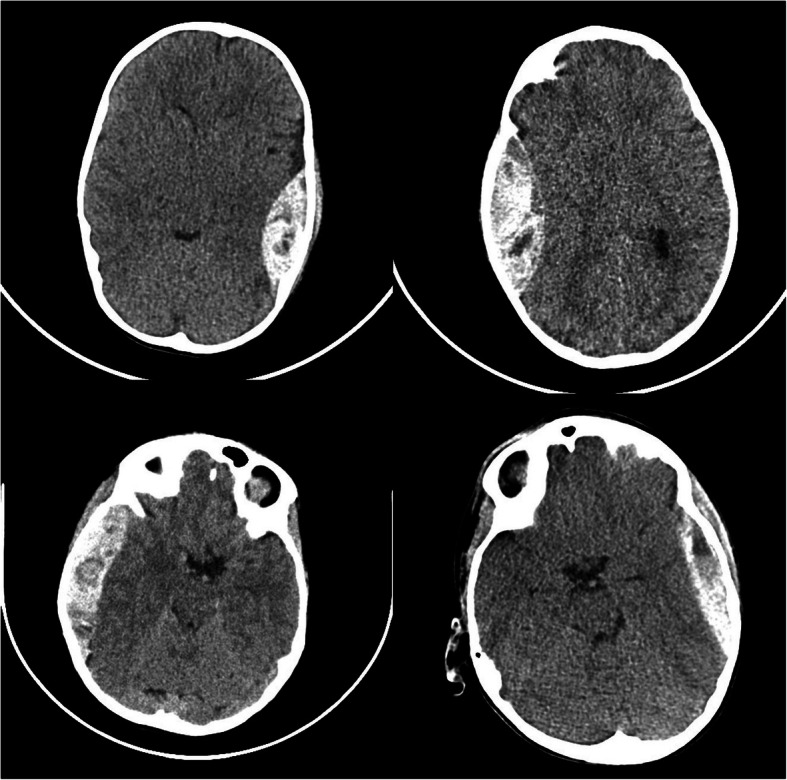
CT scans of 4 distinct cases of surgically treated EDH
